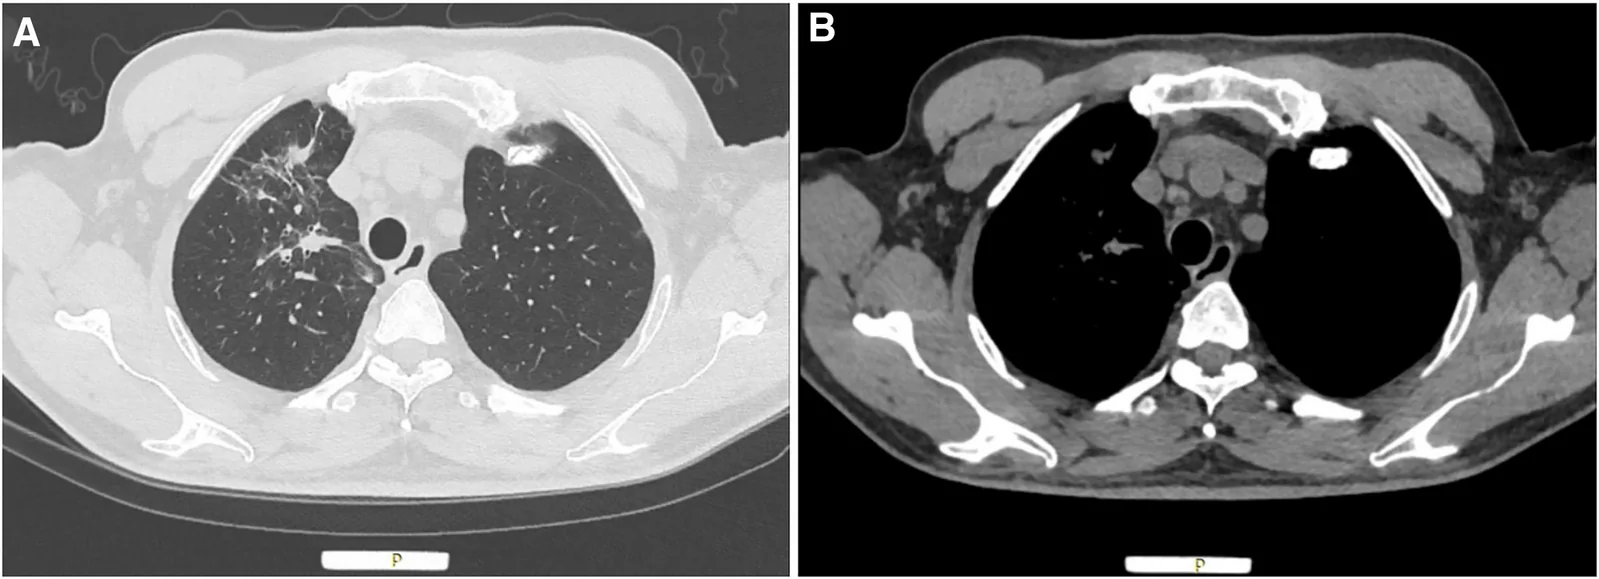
After 6 d of methylprednisolone treatment, 16-slice computed tomographic scan showed that the right upper lung lesion was significantly smaller and the cavity had disappeared. (A) Lung window; (B) mediation window.
